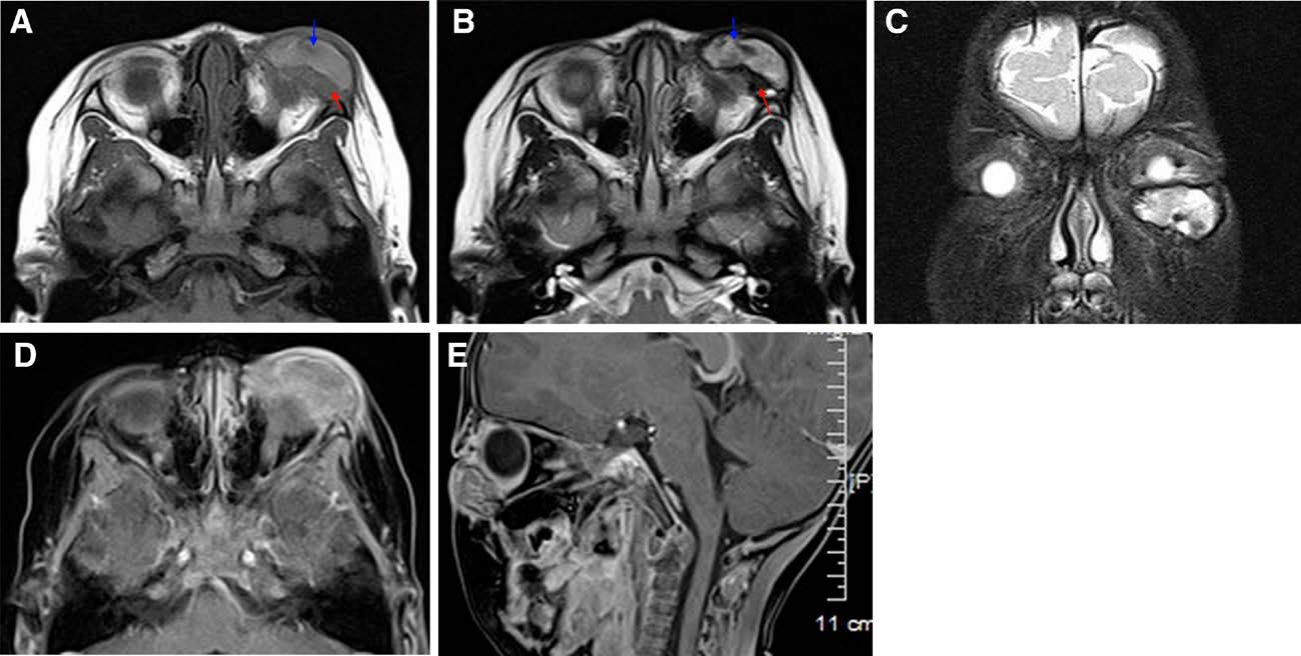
Orbital MRI revealed a well-circumscribed circular mass in the lower margin of the orbit (Blue arrow), and a second lesion under the hematoma and adjacent to the damaged bone (Red arrow). (A) Axial MRI scan on T_1_WI; (B) Axial MRI scan on T_2_WI; (C) Coronal MRI scan on fat-suppressed T_2_WI; (D) Axial MRI scan on contrast-enhanced T_1_WI; (E) Sagittal MRI scan on contrast-enhanced T_1_WI. MRI = magnetic resonance imaging, T1WI = T1 weighted images, T2WI = T2 weighted images.
